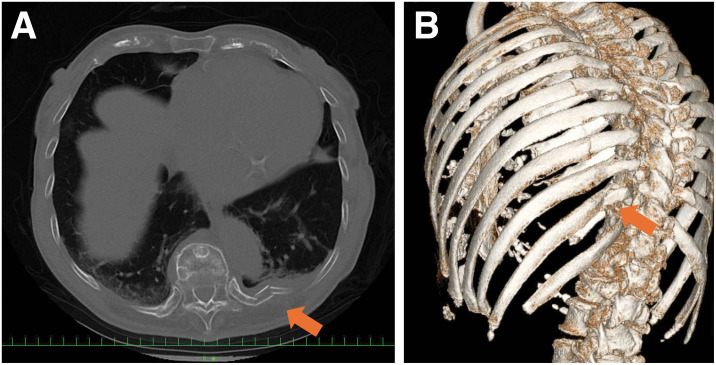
Initial CT at the referring hospital. (A) Non-contrast axial image demonstrating a medially displaced left posterior 11th rib fracture fragment adjacent to the descending thoracic aorta. (B) 3D VR reconstruction showing extensive bilateral rib fractures (left ribs 2–11 and right ribs 4–7), including the displaced posterior left lower rib fracture fragment in close spatial relationship to the descending thoracic aorta. The arrows indicate the medially displaced posterior left 11th rib fracture fragment. VR, volume-rendered
